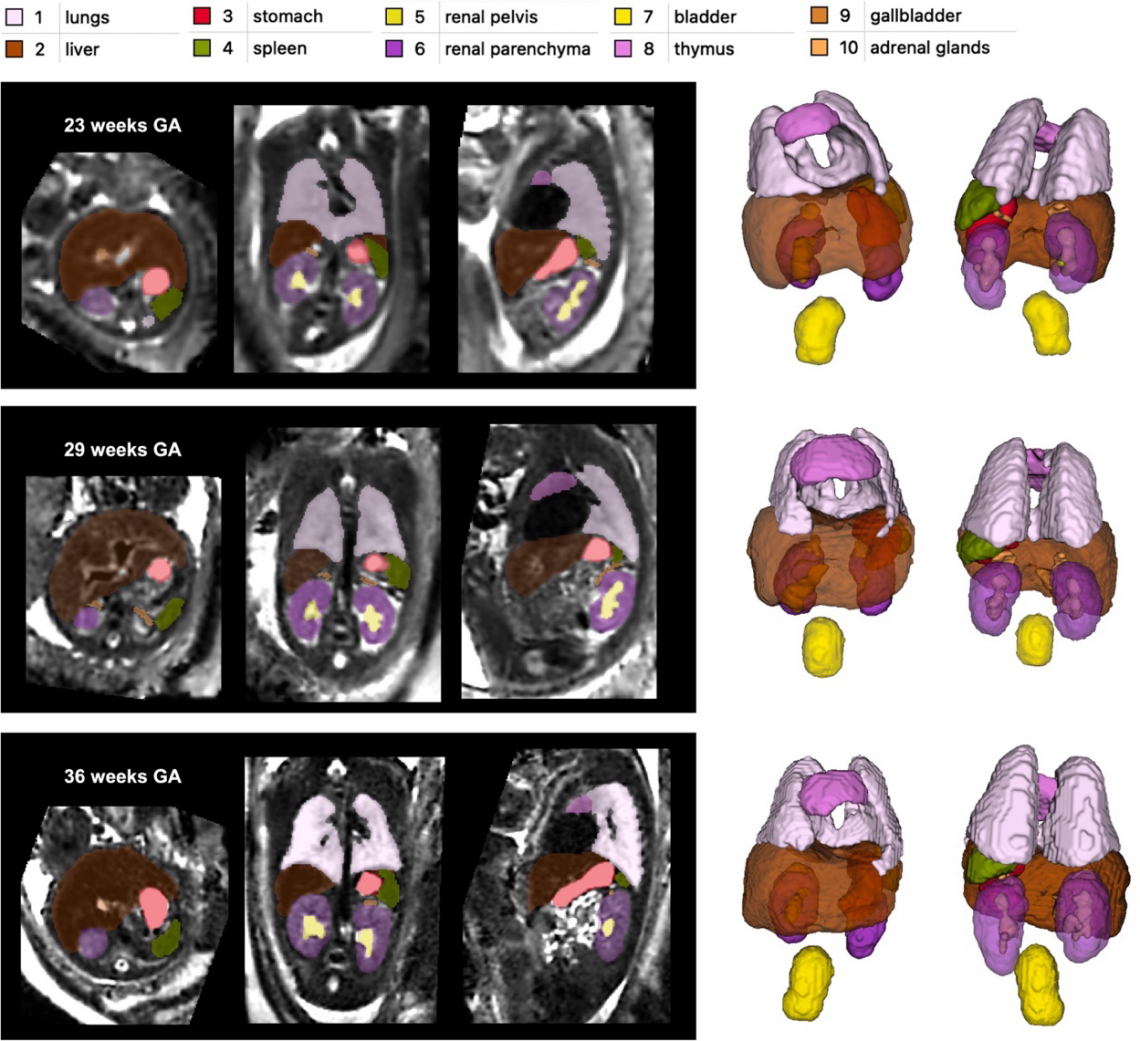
Proposed body organ parcellation protocol for 3D T2w fetal MRI. Examples at 23, 29 and 36 weeks GA.
